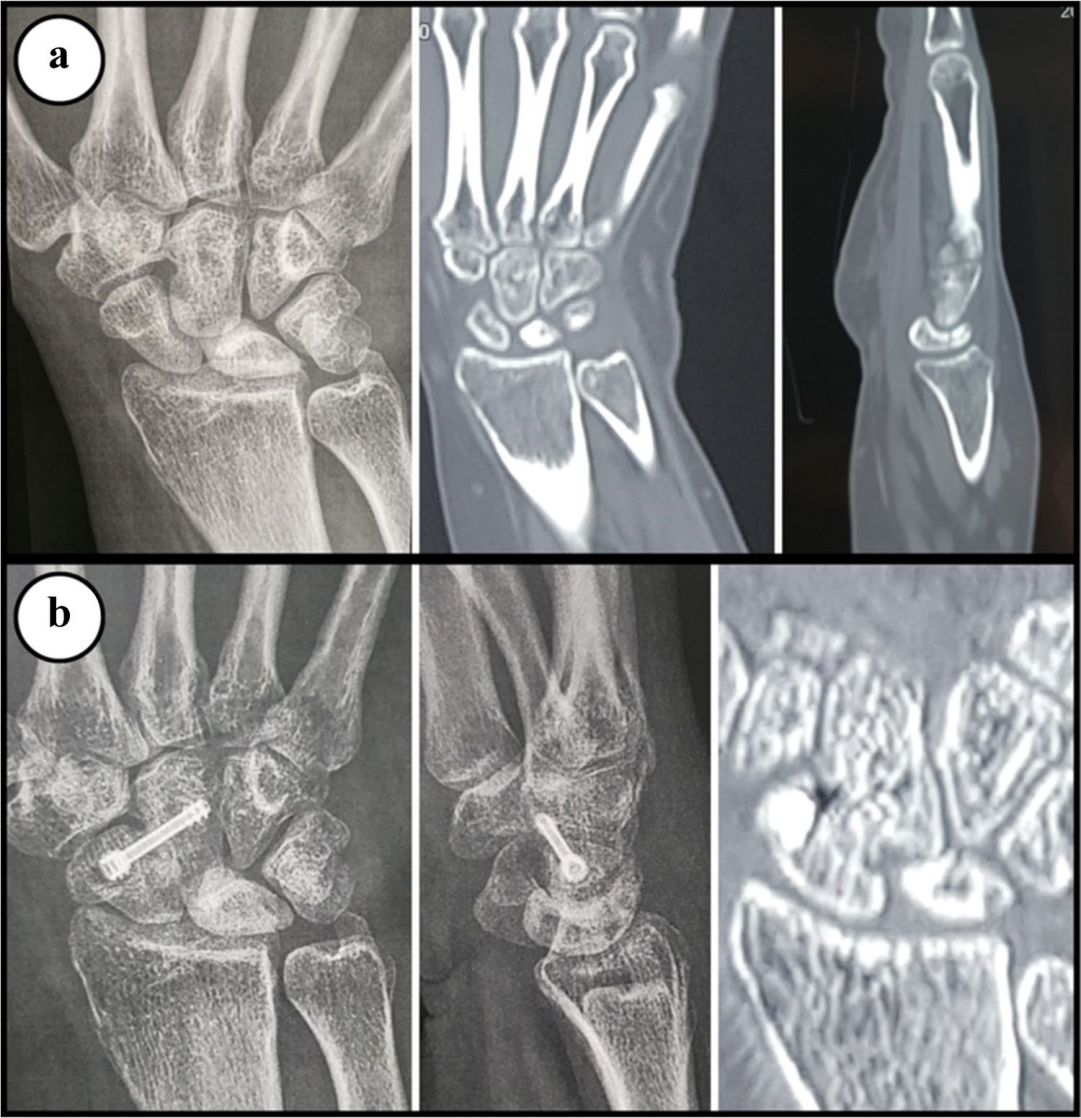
Example of radiographic images of a patient from this study. a) Pre-operative X-ray and CT scan. b) Post-operative X-ray and CT scan. Note that the trabeculae bridging the fusion line indicate fusion
